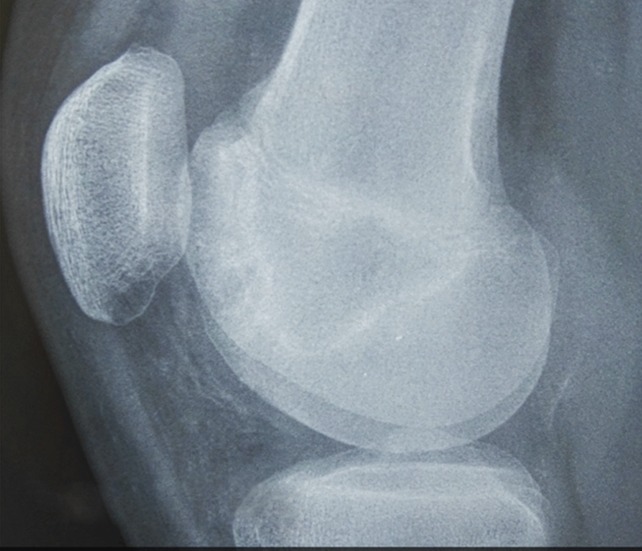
Right knee radiograph image. Lateral view. Not well-identifiable cystic lesions of the femoral trochlea.
